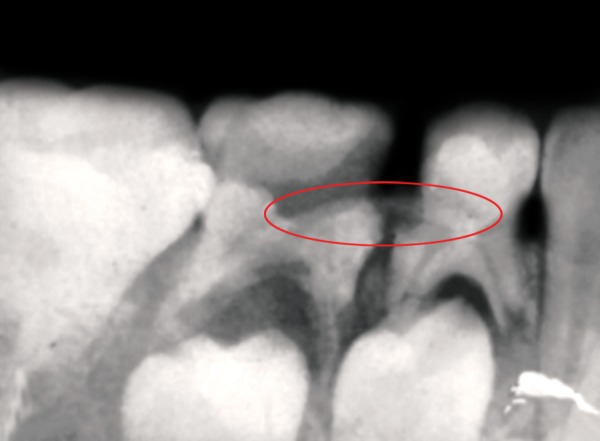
IOPA radiograph showing radiopaque object in the canals of adjacent deciduous molars
